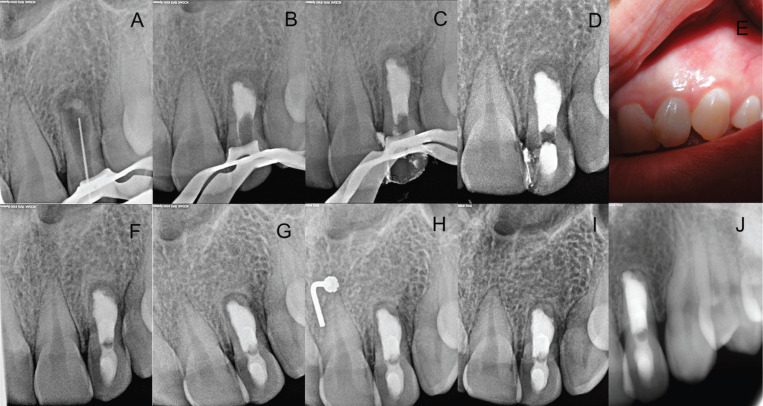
A. Working length X-ray determination; B. MTA apical plug; C. Gutta-percha backfill; D. Provisional restoration; E. Clinical follow-up at 6 months; F. Radiographic follow-up at 6 months; G. At 1 year; H. At 2 years; I. At 4 years; J. At 8 years.
